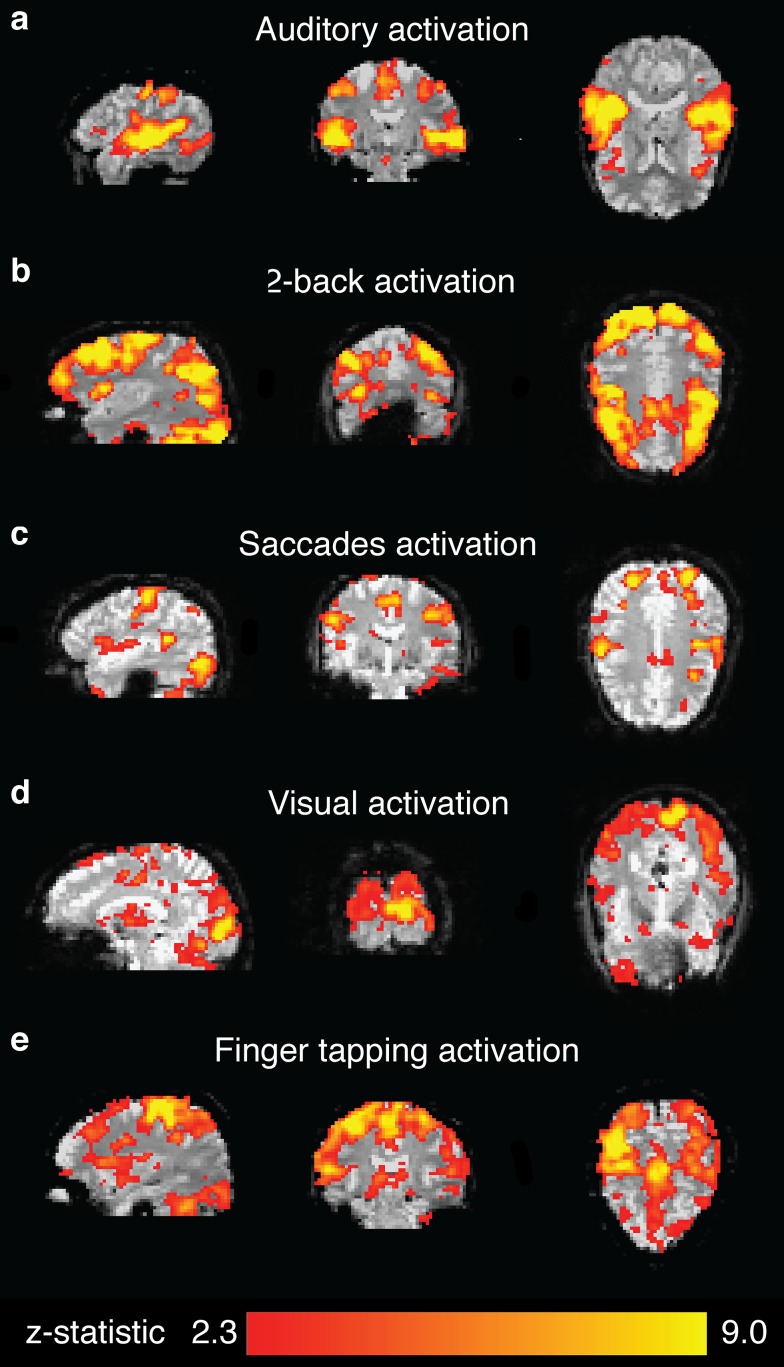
Functional MRI. Single subject Z-statistic maps showing BOLD activation for each task for the same subject as Figs. 1 and 2. Amongst other regions, the auditory task activated perisylvian primary auditory cortex (a), the working memory task activated DLPFC (b), the eye-tracking task activated FEF (c), the visual task activated occipital visual cortex (d); and the finger-tapping task activated primary motor and somatosensory cortices (e).
